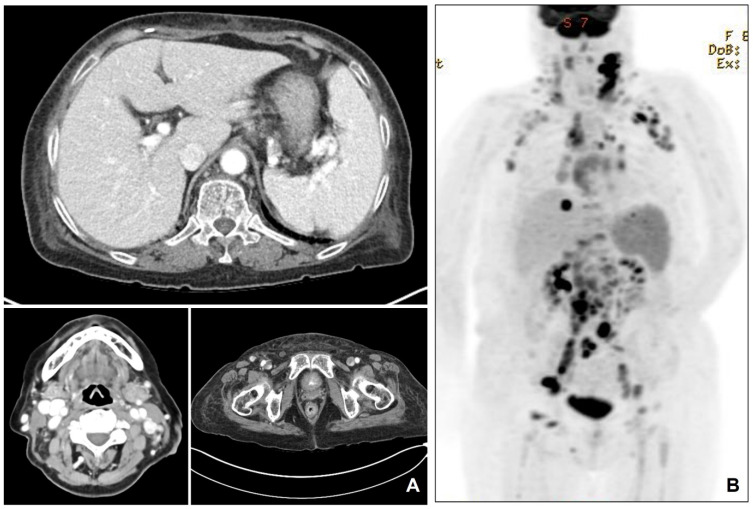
The CT scan shows splenomegaly, and multifocal hypervascular lymphadenopathy, including neck, abdomen, and inguinal area (A). The PET-CT shows multifocal involvement of lymph nodes, spleen and bone marrow (B).
